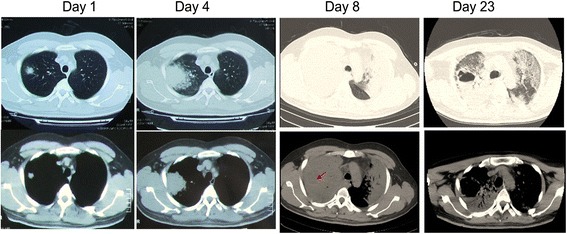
Dynamic changes on computed tomography scans for human adenovirus type 55 pneumonia in patient 3. Chest computed tomography scans taken on day 1 show a nodular shadow in the right upper lobe. The nodular shadow expanded dramatically within 3 days and was surrounded by ground-glass opacity on day 4. The lesion was diffuse in both lung fields on day 8. A cavity was observed on day 23, and the mediastinum window shows the formation of pulmonary abscess in the upper right lobe (red arrow). The lung abscess tested negative for *Legionella*, *Staphylococcus* and tuberculosis.
